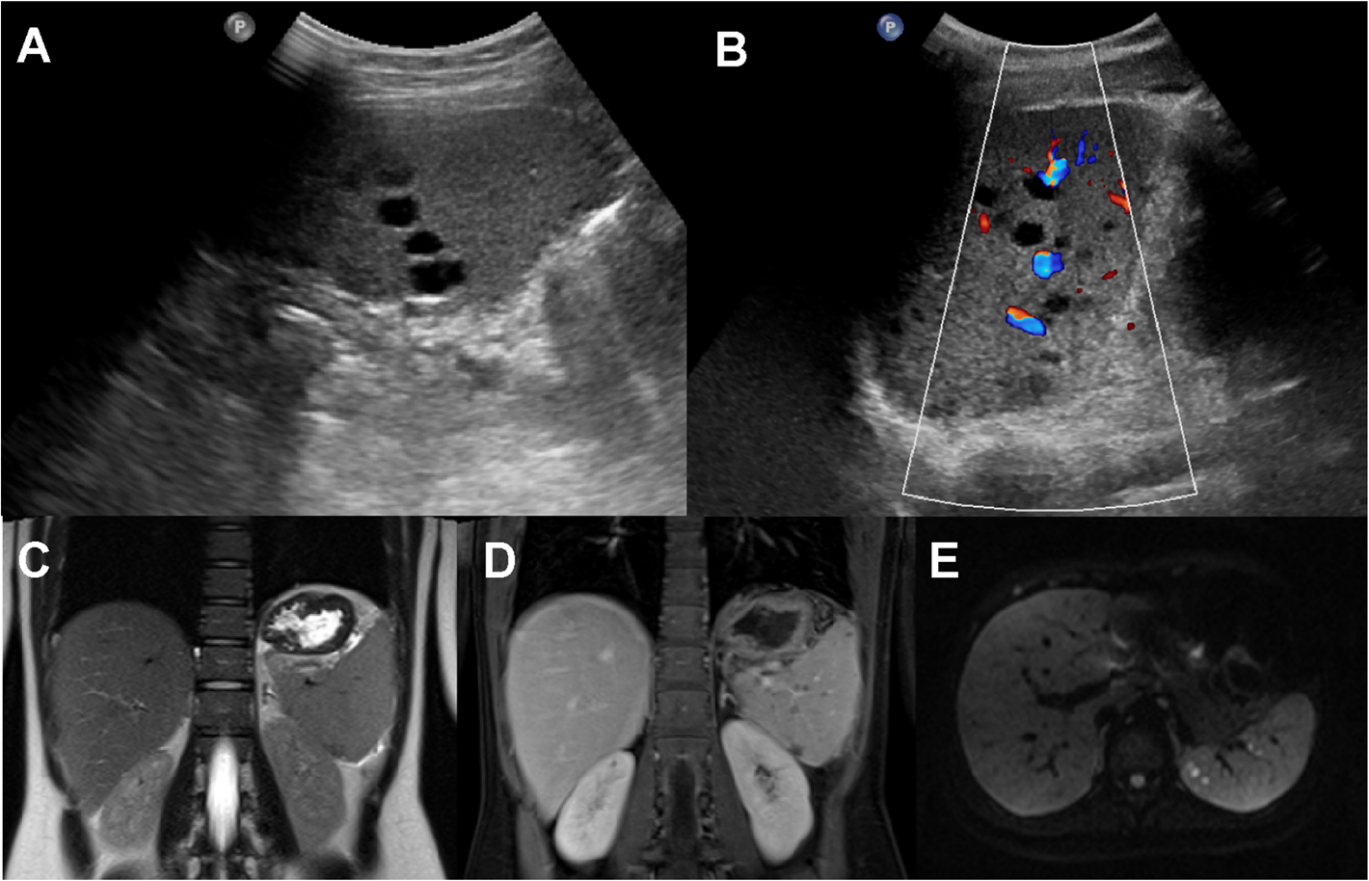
Initial imaging of the abdomen. A, B. Ultrasound of the spleen showing multiple an-/hypoechogenic masses without perfusion in the duplex sonography. C-E. MRI of the abdomen. C, the coronal T2w image demonstrates slightly hypo-/isointense areas in the spleen. D, the coronal postcontrast T1w image demonstrates hypointense lesions in the spleen, without contrast enhancement. E, the lesions show restricted diffusion on the transverse DW image (b 800 sec/mm^2^).
